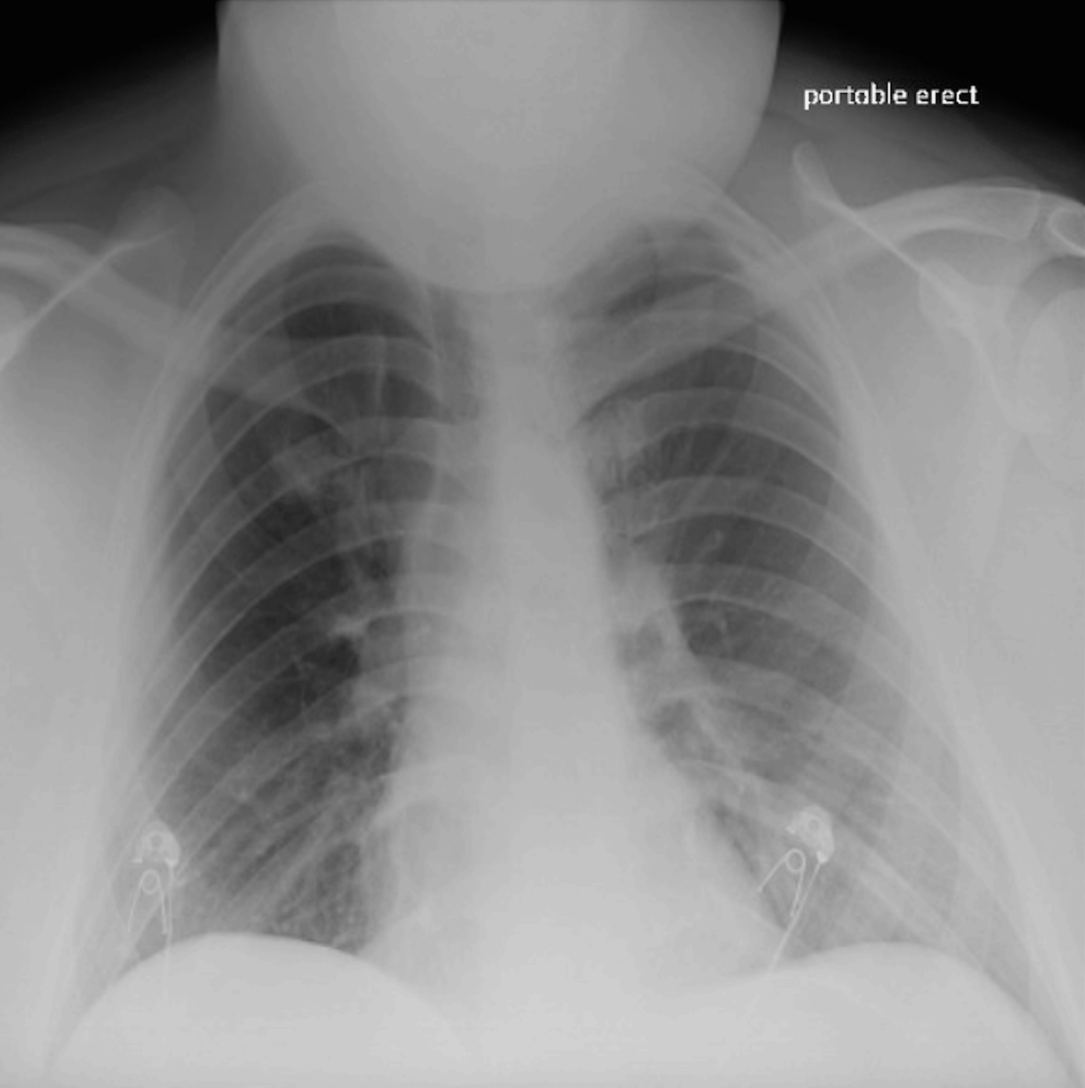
The chest radiograph is unremarkable, with no significant findings.
